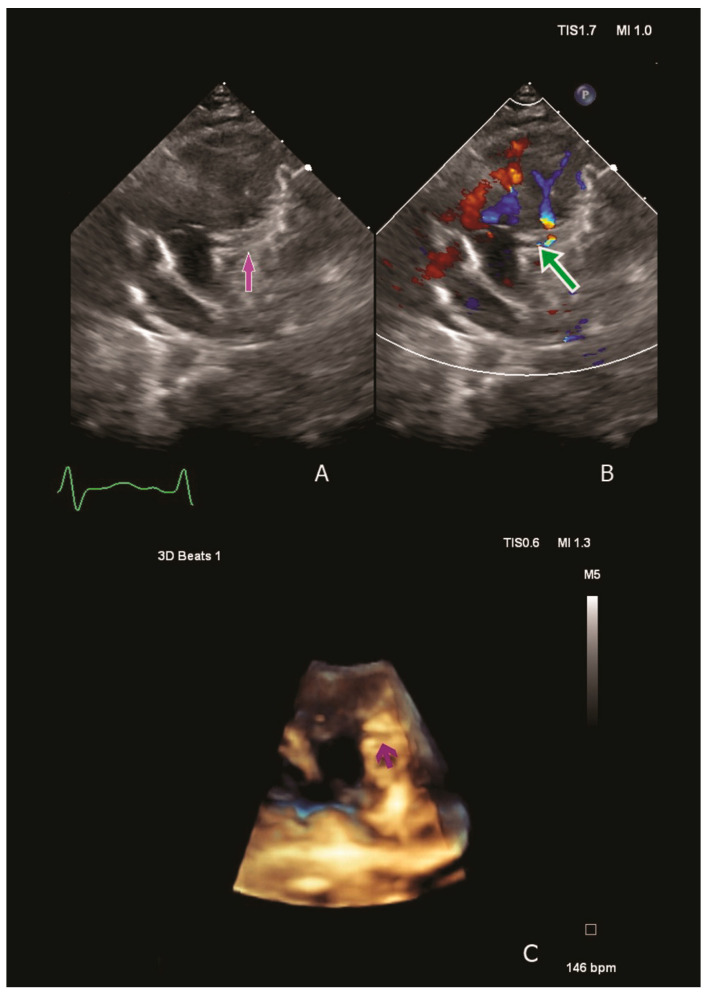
Two/three-dimensional echocardiography confirming ALCAPA diagnosis. We visualise the origin of the left coronary artery from the pulmonary artery: the purple arrow (A,C) and colour Doppler flow confirming the retrograde flow from the pulmonary artery into left coronary artery- the green arrow (B).
